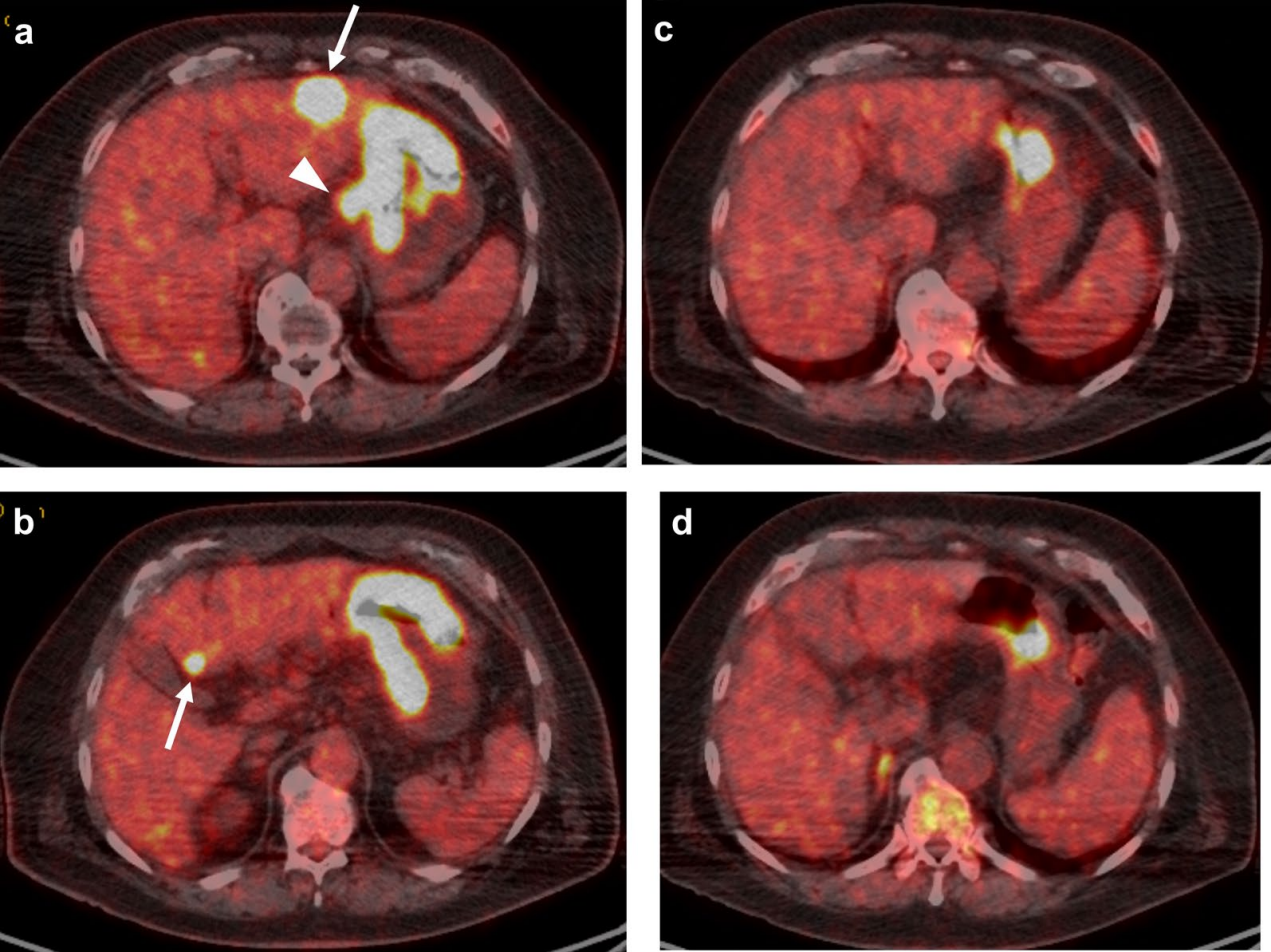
Positron emission tomography–computed tomography. a, b Before chemotherapy. Abnormal uptake in the primary tumor, enlarged station 3 lymph nodes (arrowhead), and tumors in the segments 3 and 4 of the liver (arrows) are seen. c, d After six courses of ramucirumab plus paclitaxel, the abnormal uptake in the lymph node and liver metastases disappeared
